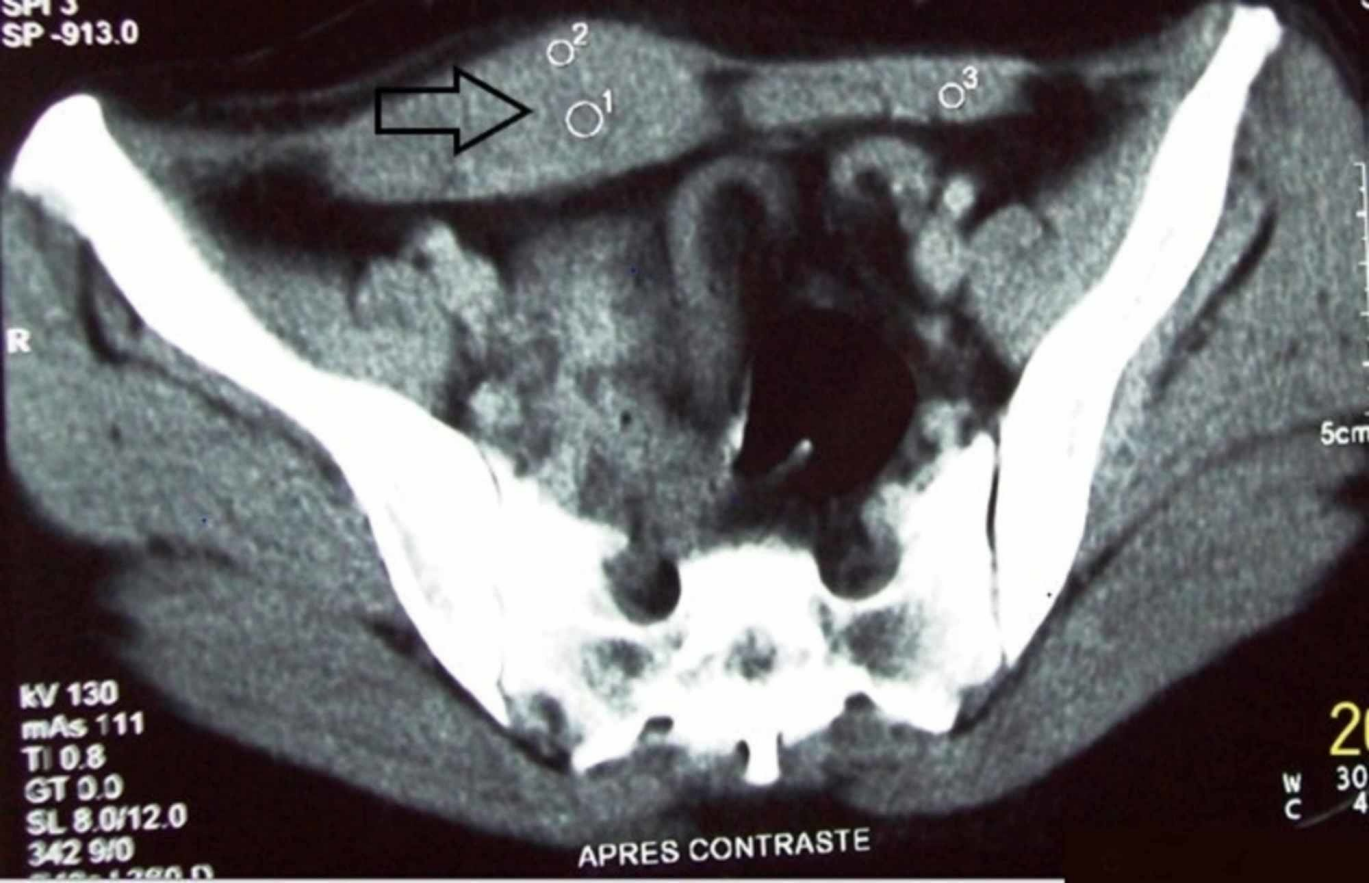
Computed tomography scan showing the endometrioma (black arrow) contained in the rectus abdominis muscle
